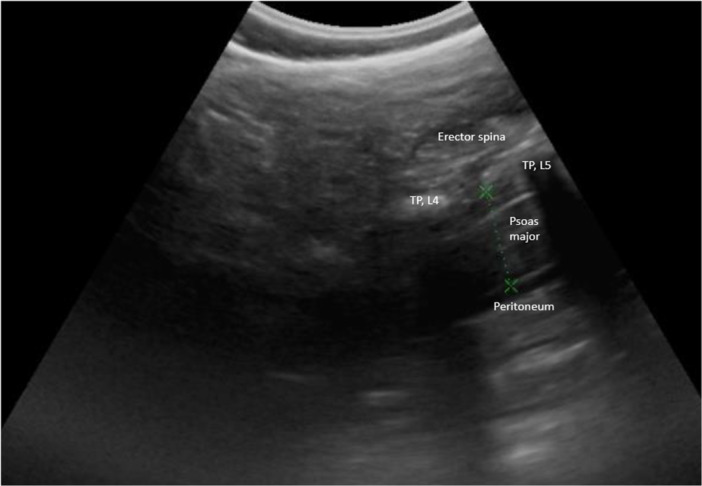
Longitudinal ultrasound imaging of the psoas major muscle at the L4–L5 level during thickness assessment.
